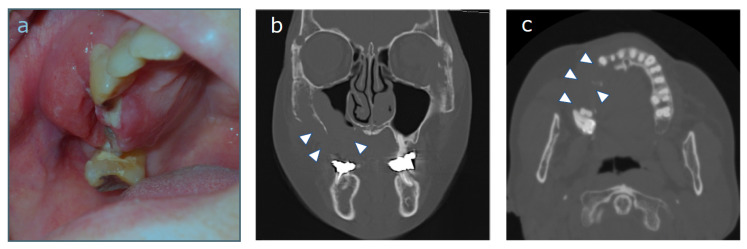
(a) An intraoral view at the first examination. A localized, well-defined swelling of size 4 cm × 5 cm in its greatest dimensions was obliterating the buccal and palatal vestibules in the region of the unhealed extraction sockets of 14, 15 and 16. Coronal (b) and axial (c) computed tomography imaging showed the osteolytic focus in the maxilla (white arrowhead).
